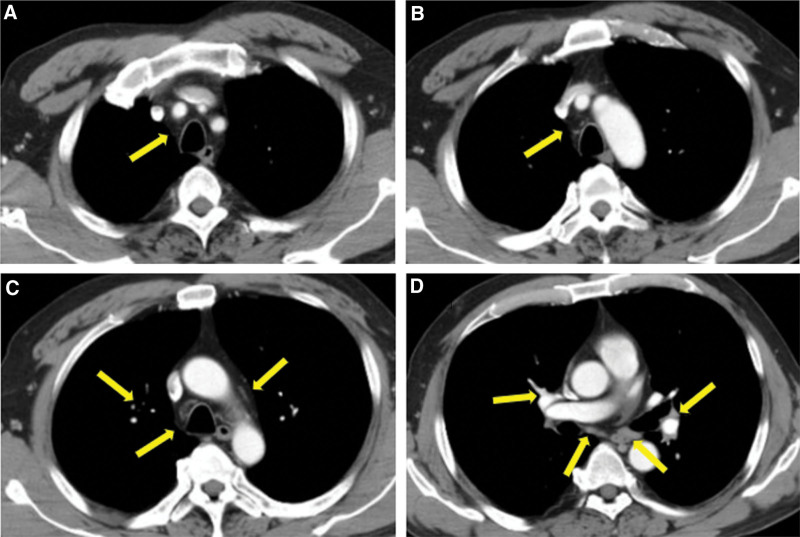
Computed tomography scan after two months of the administration of corticosteroid demonstrating the enlargement of lymph nodes shrinkage (yellow arrows).
